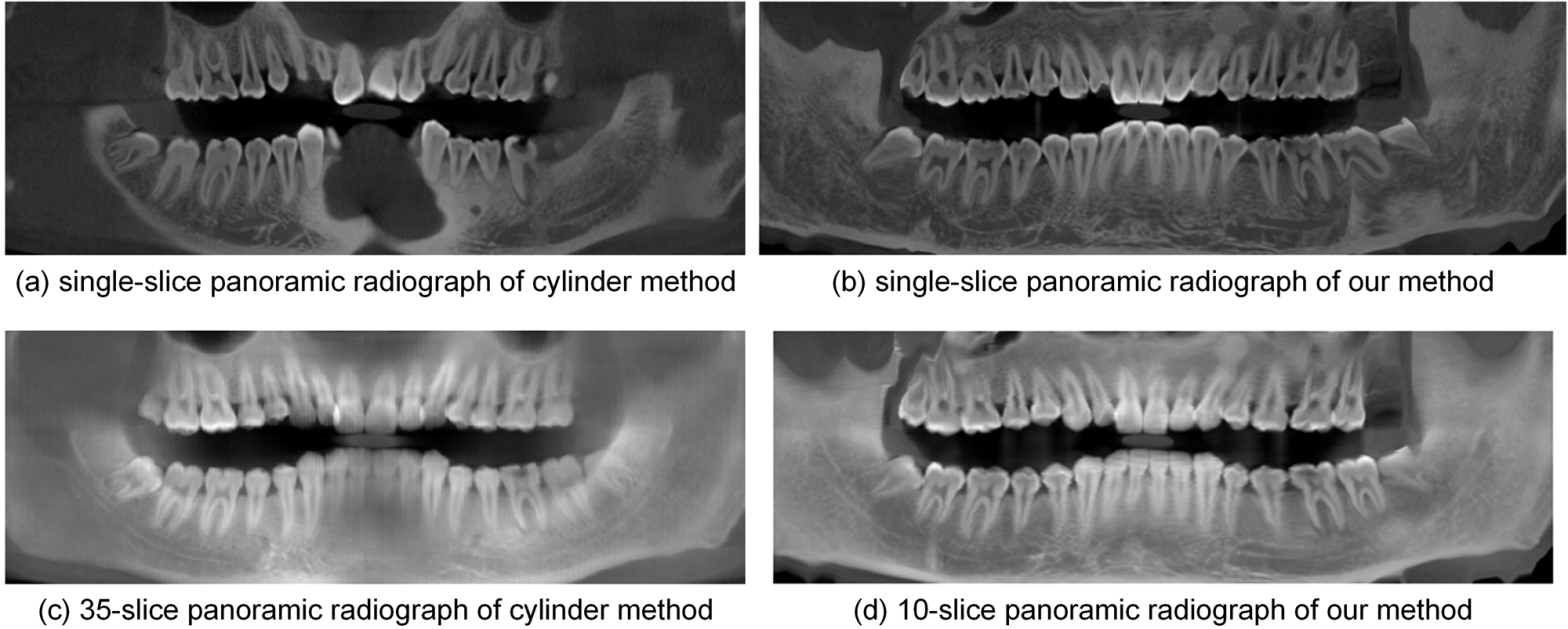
Panoramic radiographs by different methods from dataset 3.
